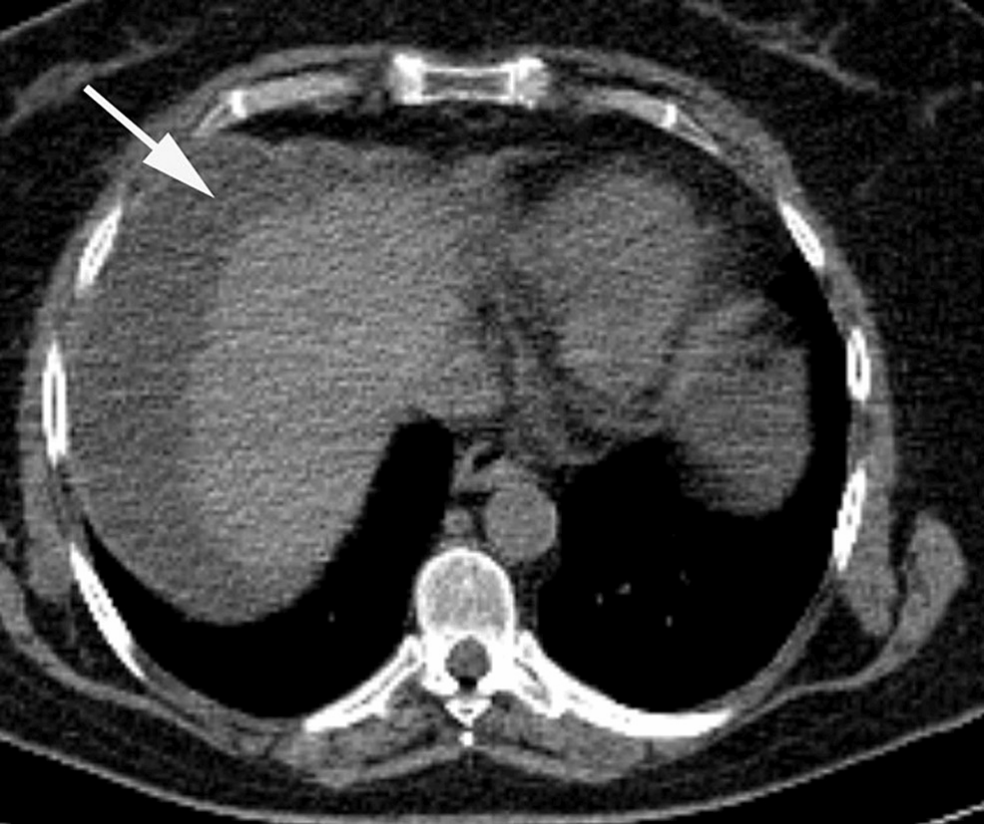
Non-contrast enhanced CT of the upper abdomen Upper abdominal incidental findings on computed tomography CT images, ascites (arrow)
